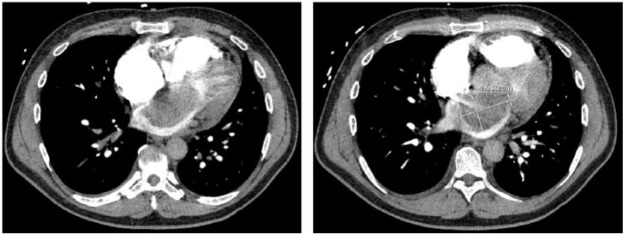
CT chest of a large left atrial myxoma. Dimensions of the LA mass are 6.1 cm × 4.5 cm. We could not determine whether the LA mass is a LA myxoma or a large thrombus on chest CT scan.
